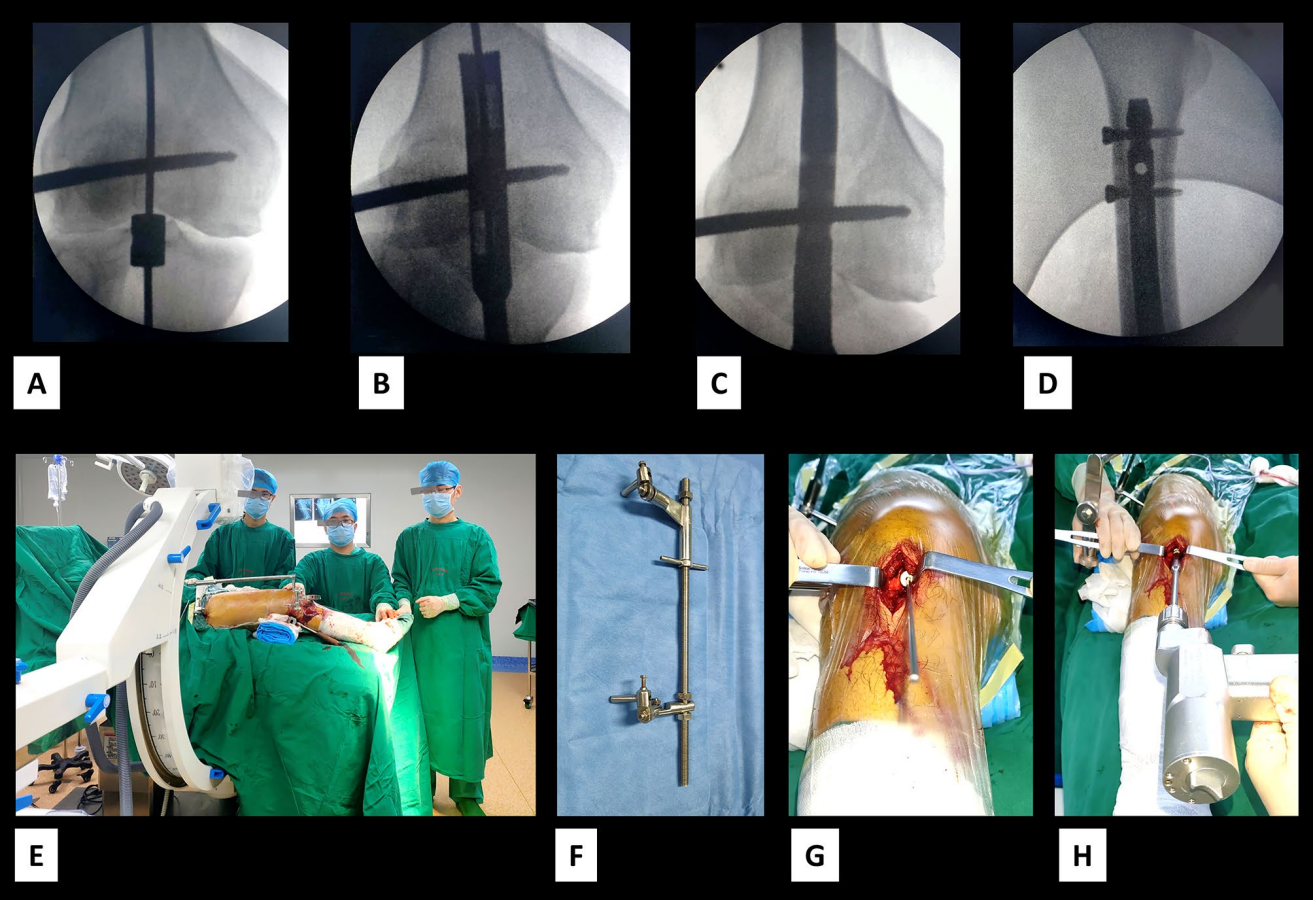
The hollow trephine can also be used in unilateral retractor-assisted surgery A-D: Anteroposterior and lateral radiographs showing intraoperative application of the hollow trephine E, F: The use of a unilateral retractor. (the images provided in Fig. 3E are authors images) G, H: Open-hole reaming of the distal femur using a hollow trephine
